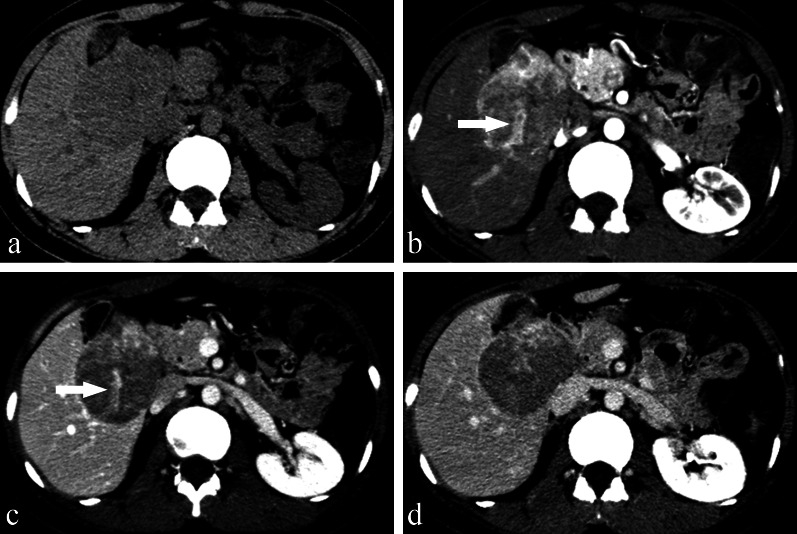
A 24-year-old female with pathologically diagnosed HEHE. a Axial unenhanced CT shows an isolated mass in the centre of the liver. Marked enhancement on the arterial phase, (b) of the whole tumour, and washout occurred quickly in the next two phases (c, d). Enlarged, persistently enhancing blood vessels are seen within the tumour (*arrows,*
